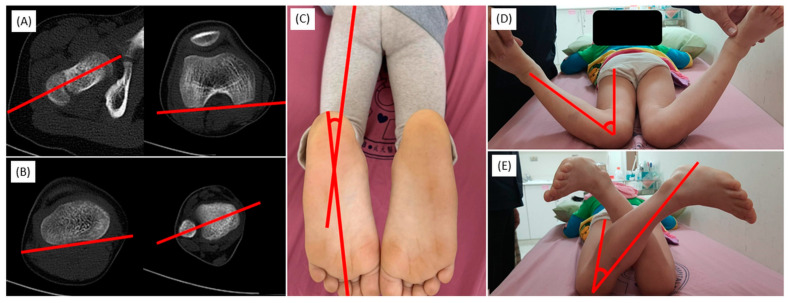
CT scans demonstrating femoral anteversion (A), tibial internal torsion (B), with clinical evaluation of thigh–foot angle (C), hip external rotation (D) and internal rotation (E).
